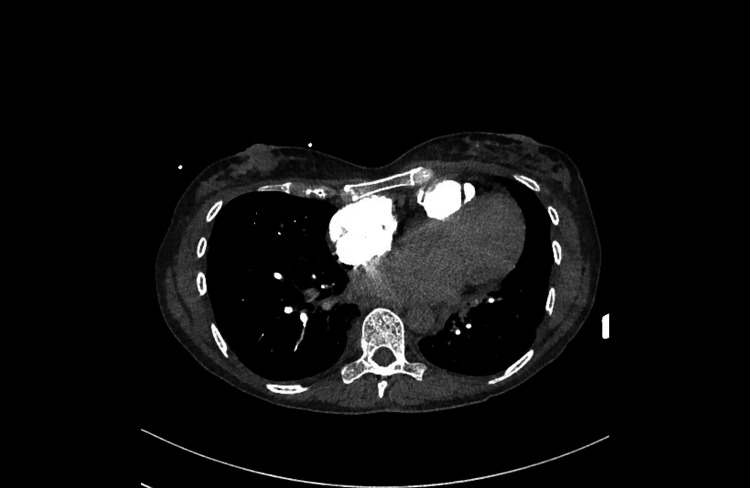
CT pulmonary angiogram showing negative for pulmonary embolism
